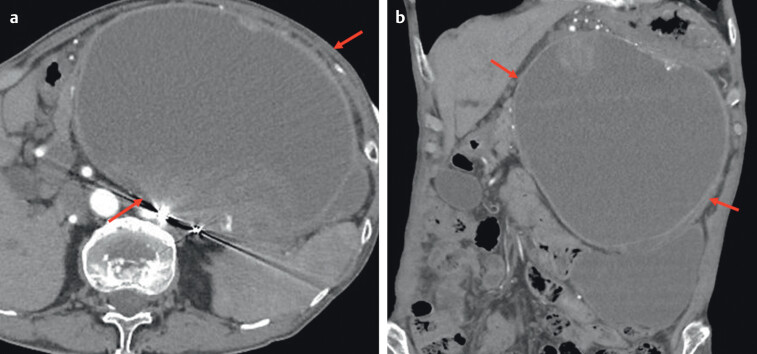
Computed tomography revealing pancreatic fluid collection
